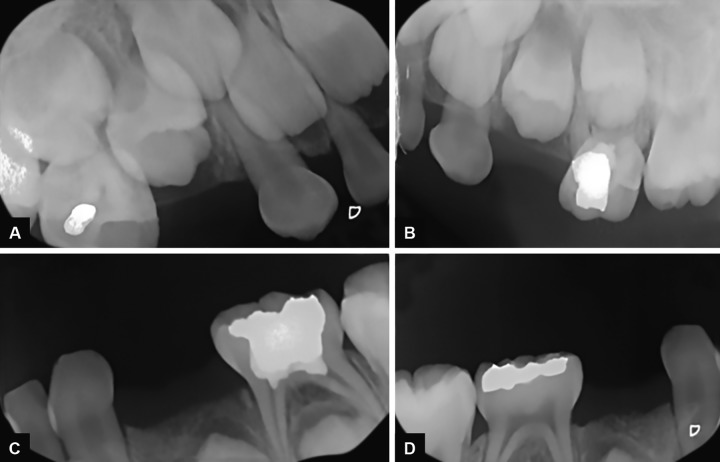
Radiographic situation 3 months after therapy
